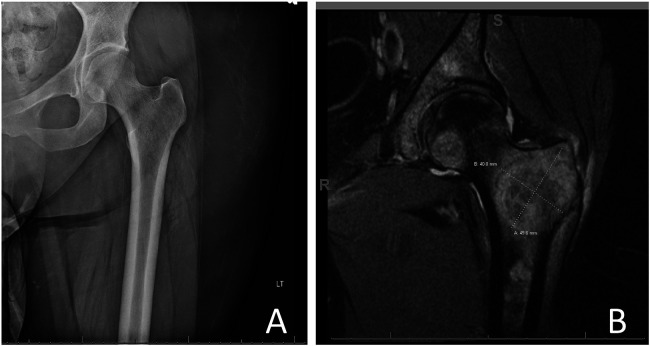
(A) Plain film x-ray of the left hip and (B) T1-weighted MRI of the left hip showing a large left hip lesion.
